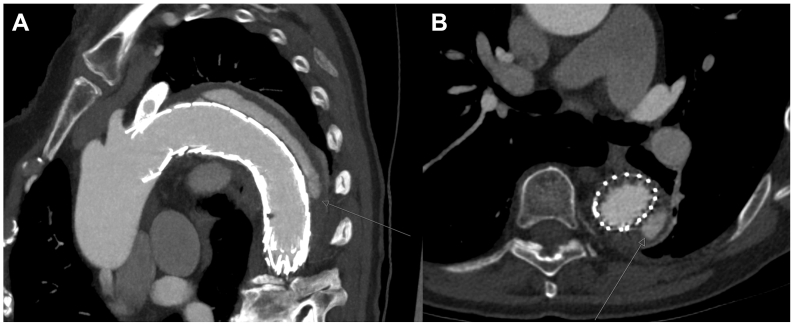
(A, B) Computed tomography angiography (CTA) demonstrating a suspected gutter-related type Ia endoleak after left subclavian artery (LSA) chimney thoracic endovascular aortic repair (TEVAR) for a complicated type B aortic dissection. The *arrow* indicates the percutaneous transthoracic access route to the false lumen.
